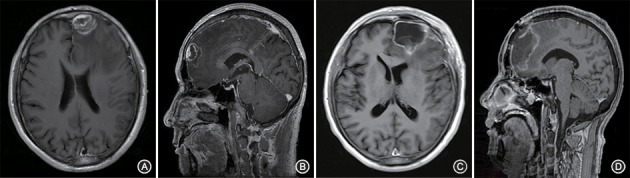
Enhanced MRI shows irregular masses lumped together mixed with high- and low-signal foci on the left frontal lobe. The border is less clear and is surrounded by a large low-signal shadow. The adjacent brain parenchyma is compressed, the lateral ventricle is compressed and deformed, and the central structure is slightly right-biased (A,B). At the follow-up 10 days after surgery, the left frontal lobe still presented with an annuliform high signal, the anterior border seemed to have a high nodular signal, and the lateral ventricle was still compressed and deformed (C,D).
